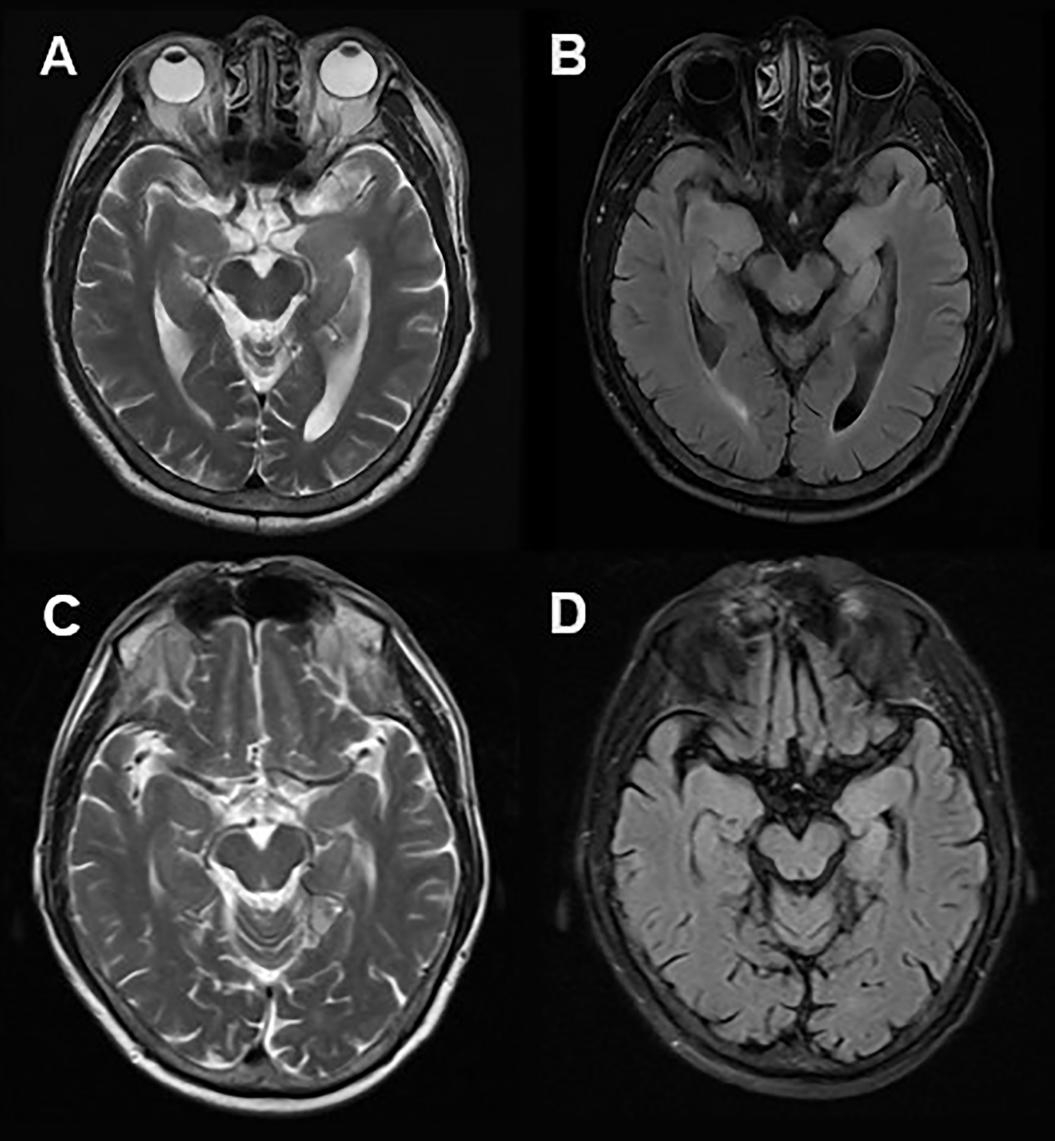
Cranial MRI in Case 3. Axial T2 weighted (A) and FLAIR (B) sequences showed hyper-intensity in the left hippocampus. 2 months after immunotherapy, a control cranial MRI showed a significant reduction of right hippocampus hyper-intensity in T2 weighted (C) and FLAIR (D) sequences.
